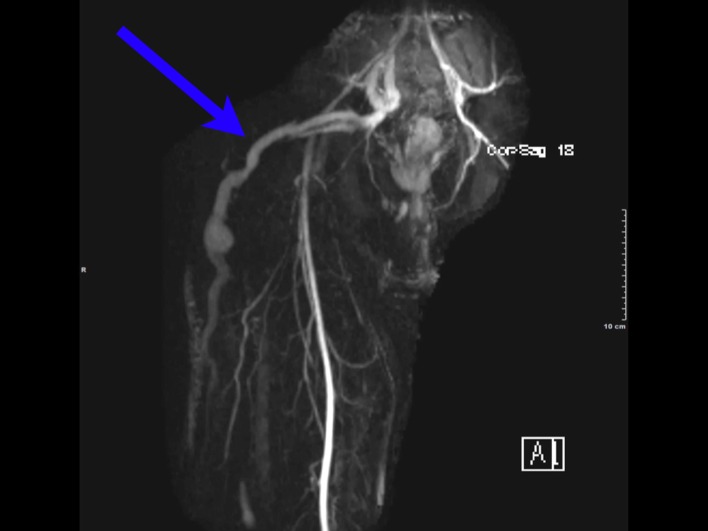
An MRI/MRV revealed an enlarged fetal lateral limb-bud vein extending into the right lateral thigh.
